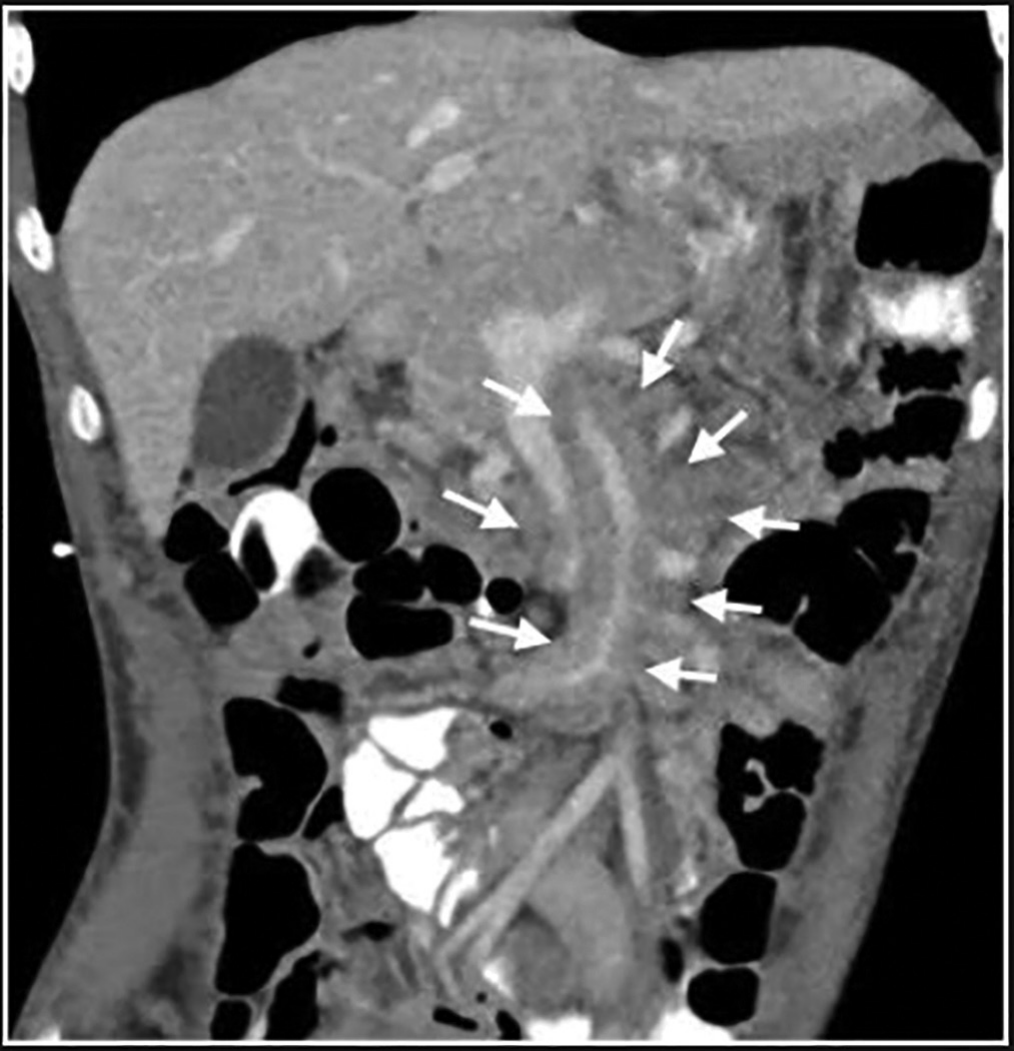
Coronal image from contrast-enhanced abdominal computed tomography shows abnormal diffuse soft tissue infiltration (arrows) throughout the mesentery, particularly around the mesenteric vessels.
